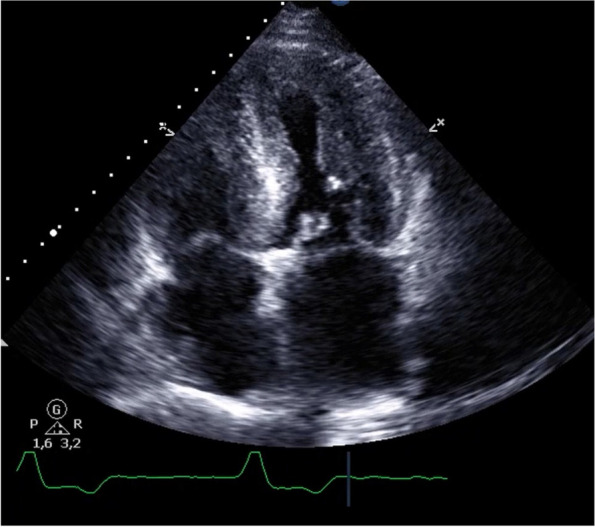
The patient’s echocardiography. Four-chamber view with prominent left-ventricular hypertrophy and granular sparkling typical for amyloidosis
